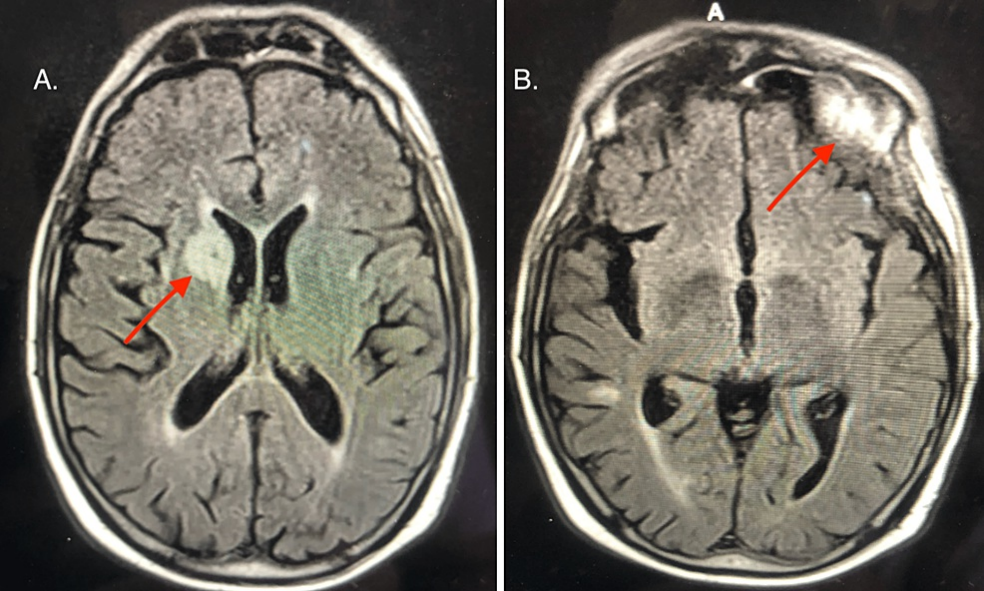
MRI with contrast revealed multiple enhancing intracranial lesions with leptomeningeal enhancement at the posterior fossa consistent with CNS metastasis 1A shows a paraventricular mass while 1B shows a mass in the left frontal lobe.
